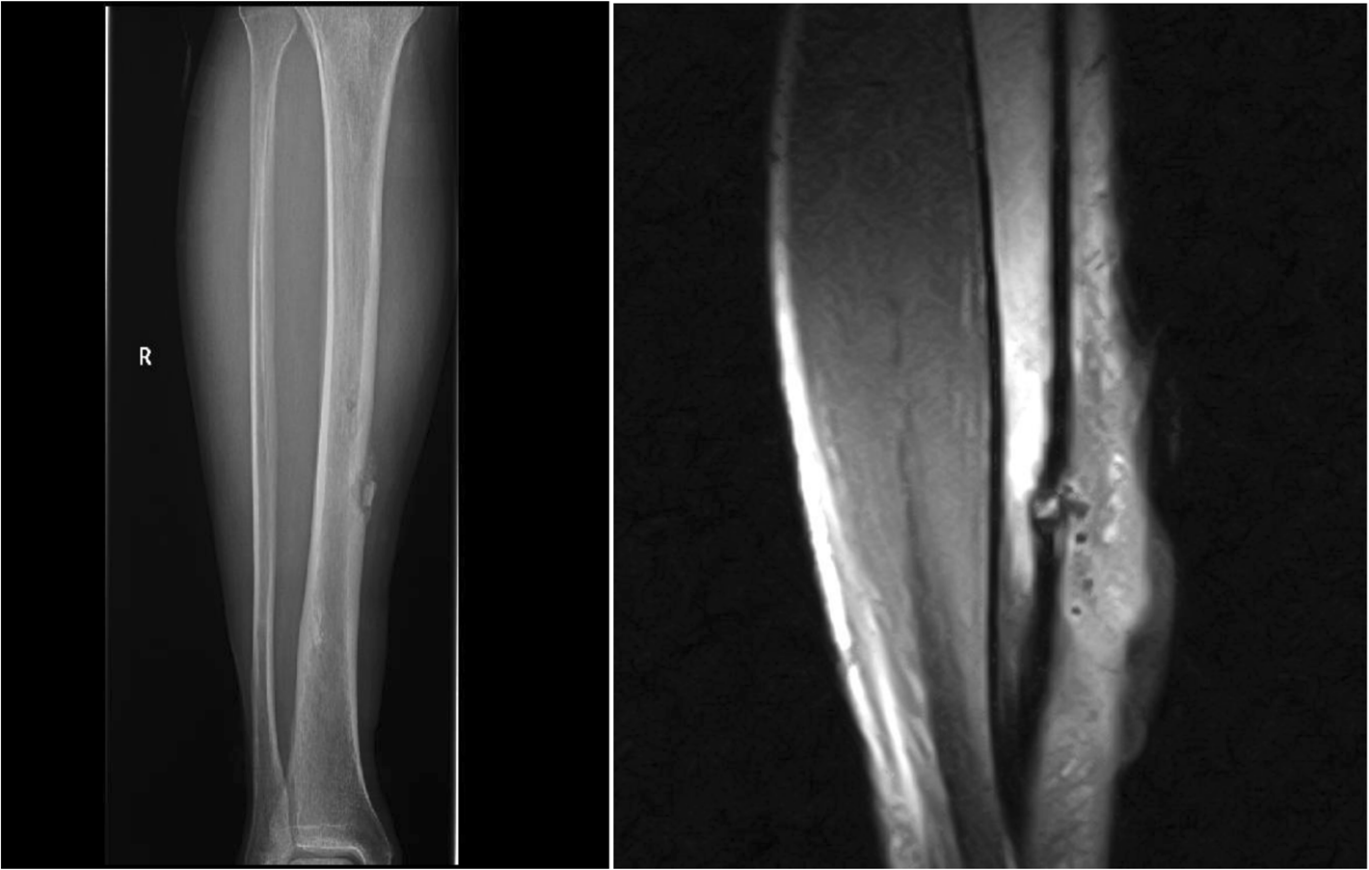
A lesion resembling osteomyelitis on a computed tomographic scan of the left lower limb
